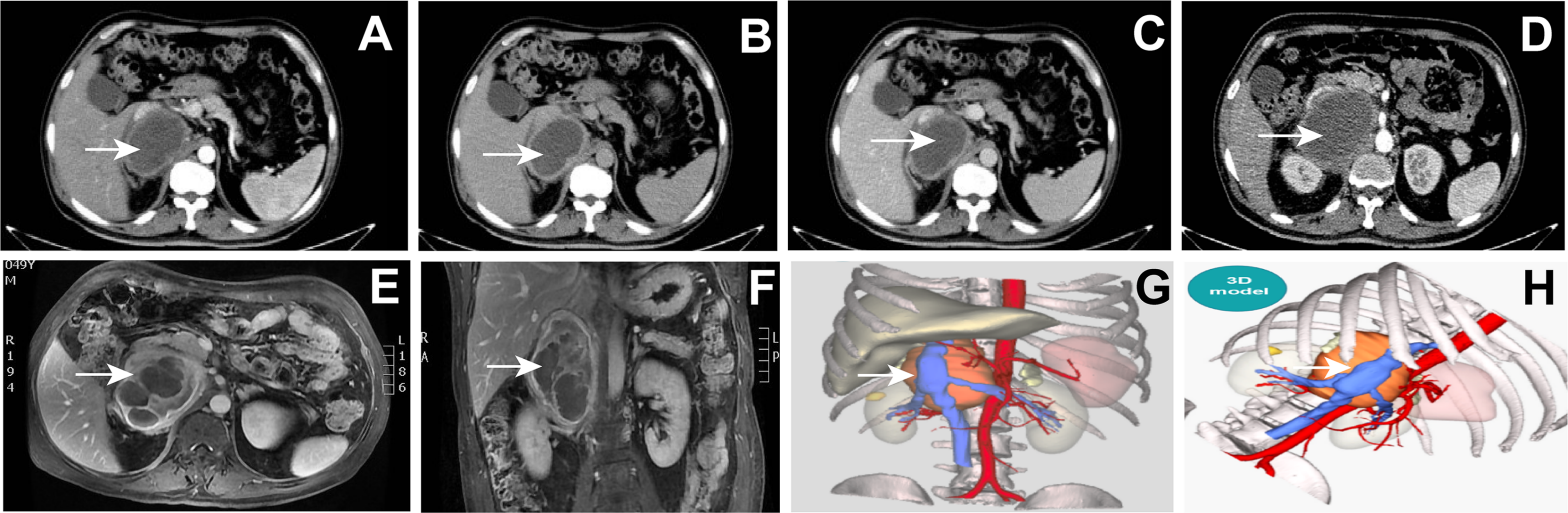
(A–D) Abdominal plain CT: right retroperitoneal cystic solid space-occupying lesion (arrow). (E–F) Magnetic resonance image of the abdomen with gadolinium contrast showing a 9.0* 6.5 * 10.7 cm right adrenal mass (arrow). (G, H) The 3D model of the abdominal lesion (arrow).
